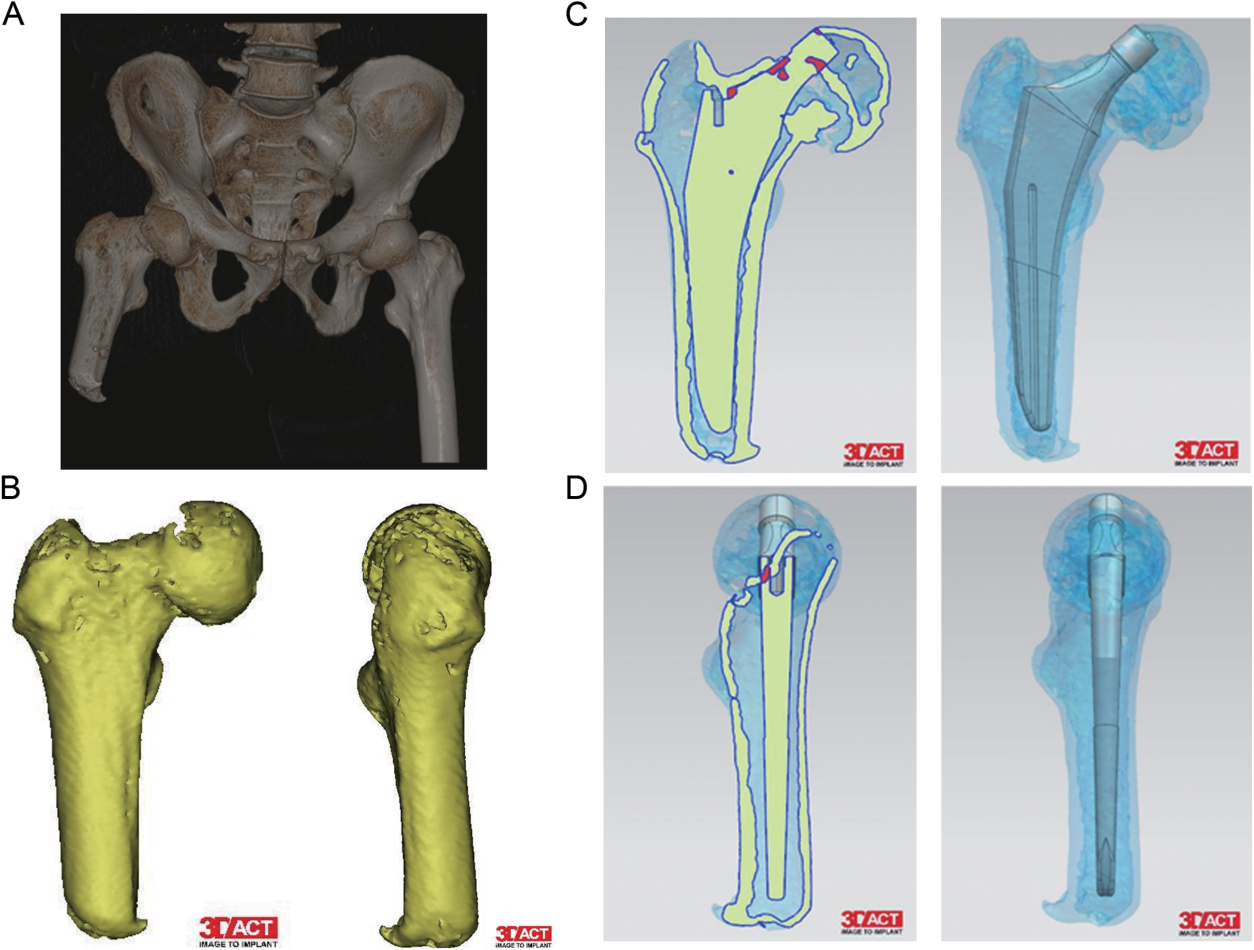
Preoperative CT 3D reconstruction and preoperative planning image. (A) CT 3D reconstruction of the pelvic. (B) CT 3D reconstruction of the right femur. (C,D) Preoperative planning images of the femur.
